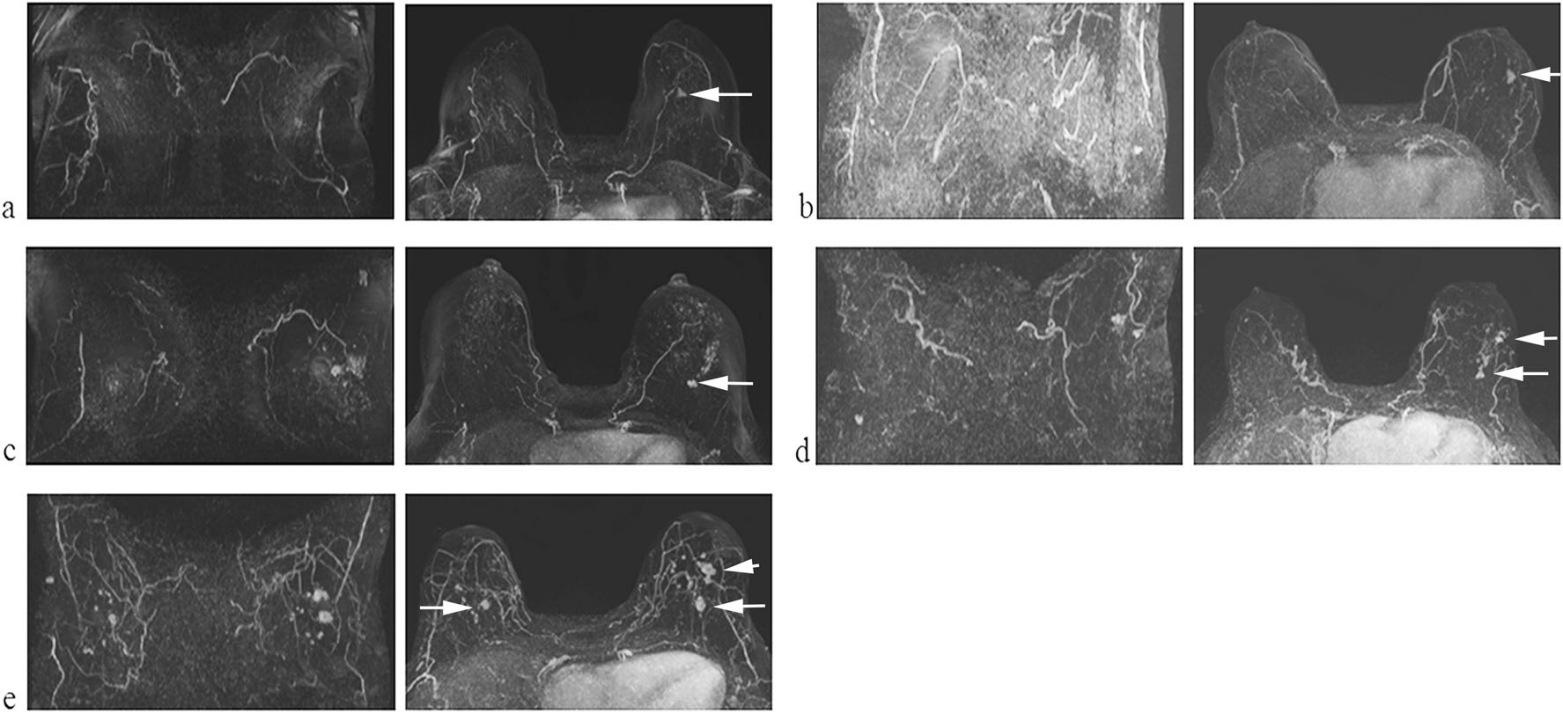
Maximum intensity projection of contrast-enhanced MRI of the 5 women with mammography-negative, 3DIRI-positive malignancies. The arrows indicate tumor. (a) 68-year-old woman with 11-mm invasive carcinoma in the left breast, (b) 73-year-old woman with 7-mm ductal cancer in situ in the left breast, (c) 56-year-old woman with 7-mm invasive ductal carcinoma in the left breast, (d) 72-year-old woman with 42-mm ductal cancer in situ in the left breast, (e) 70-year-old woman with bilateral multifocal ductal carcinoma
